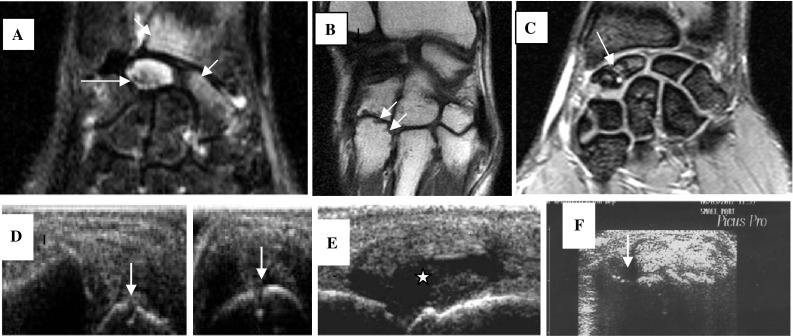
Imaging evidence of synovitis, tenosynovitis, and erosion. a Coronal STIR image shows an abnormal marrow signal involving the lunate bone (*long arrow*), also a hyperintense signal also seen partially affecting the scaphoid and the inner aspect of the radius denoting bone marrow edema (*short arrows*). b Coronal MRI image in a different patient shows erosive changes affecting the base of the 4th metacarpal bone (*arrows*). c Coronal T2 image shows erosive changes and marrow edema affecting the scaphoid bone (*arrow*). d MSUS image of bone erosion on longitudinal and transverse scan (*arrow*). e Longitudinal ultrasonographic view of MCP joint depicting grayscale synovial hypertrophy and synovitis (*asterisk*). f A transverse ultrasonographic view of the common extensor tendon at the level of the wrist showing grayscale tenosynovitis in the form of effusion (*arrow*)
